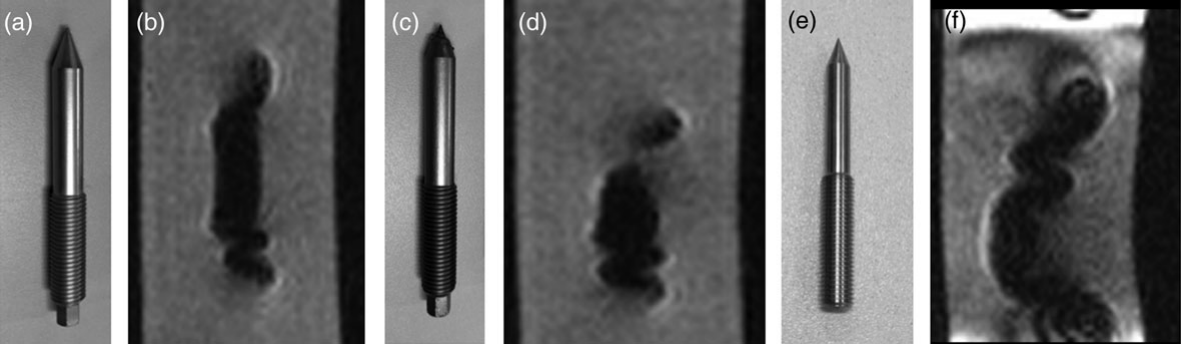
Aluminum quick fixation screw (QFS) (a), aluminum QFS with a tungsten tip (c), and titanium QFS (e), used during skull frame placement. The 3.0-Tesla MR images of each QFS embedded in gelatin (b, d, f). Distortion of the titanium QFS was remarkable. The tungsten-tipped QFS caused more distortion than the whole aluminum QFS.
